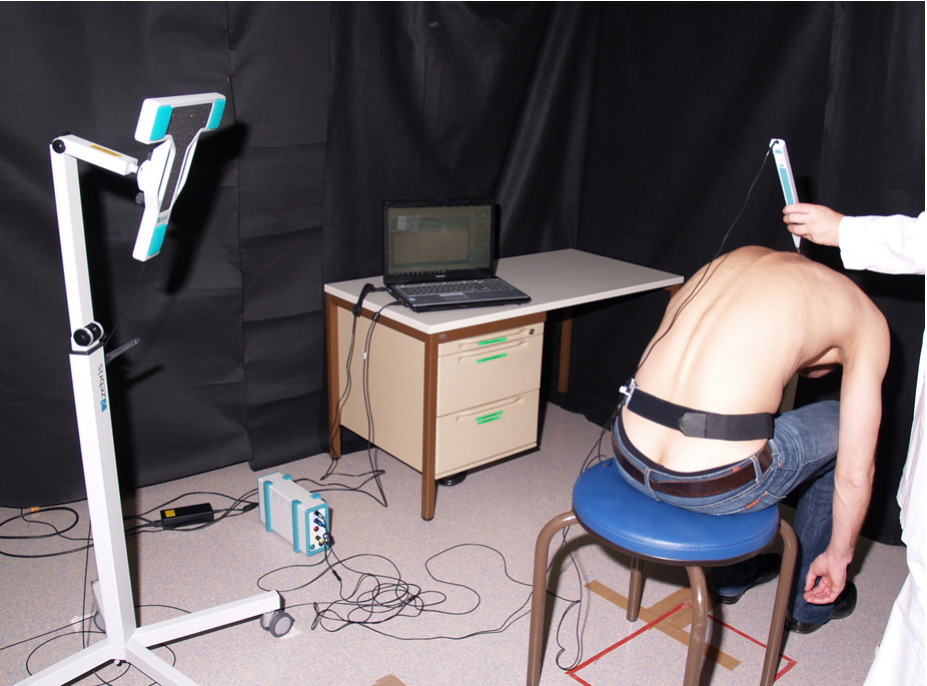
Experimental set-up of the ultrasound-based motion analysis.
